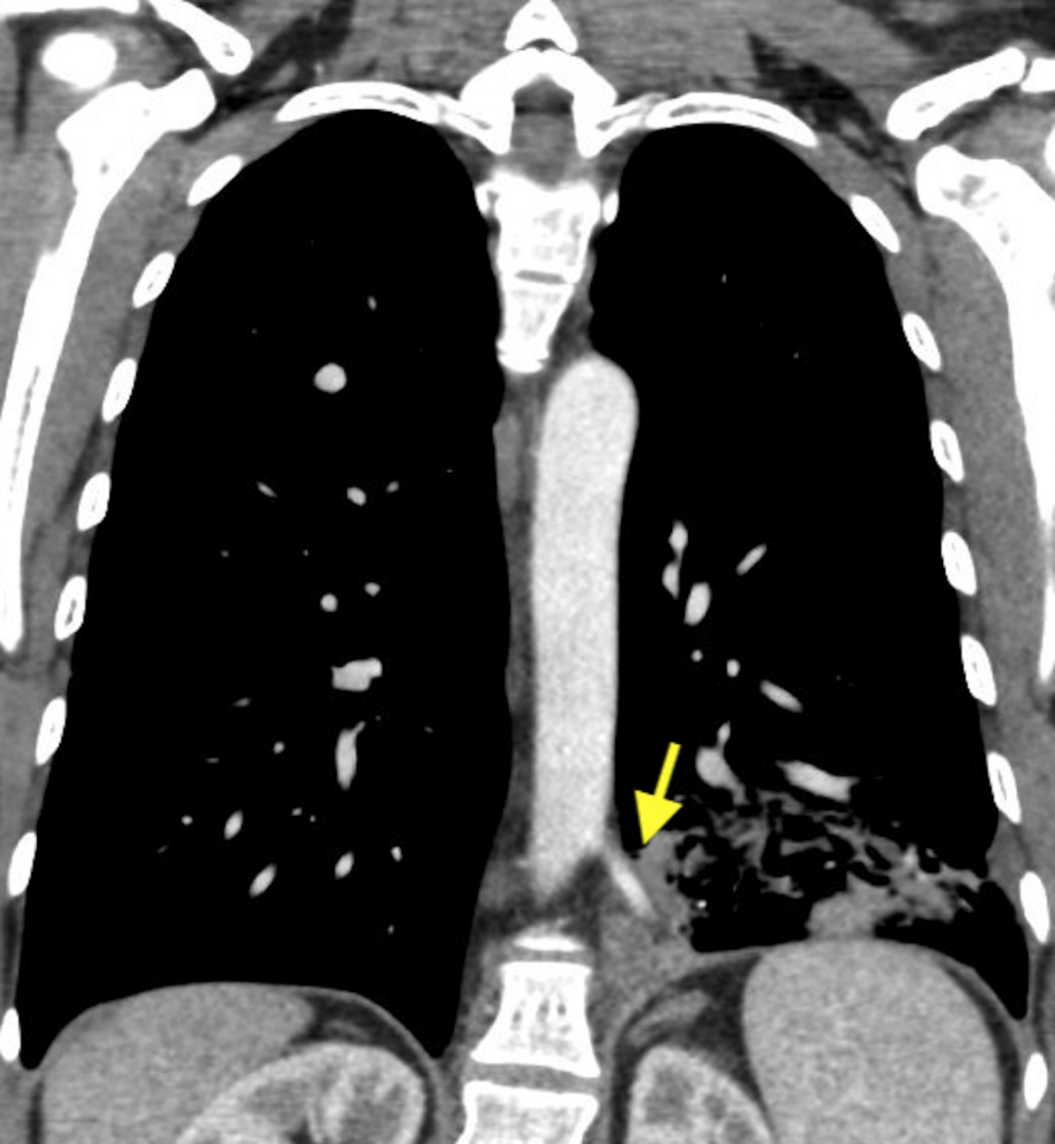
Coronal view of the CT chest with contrast. The yellow arrow shows the feeding artery entering the area of infiltration.
